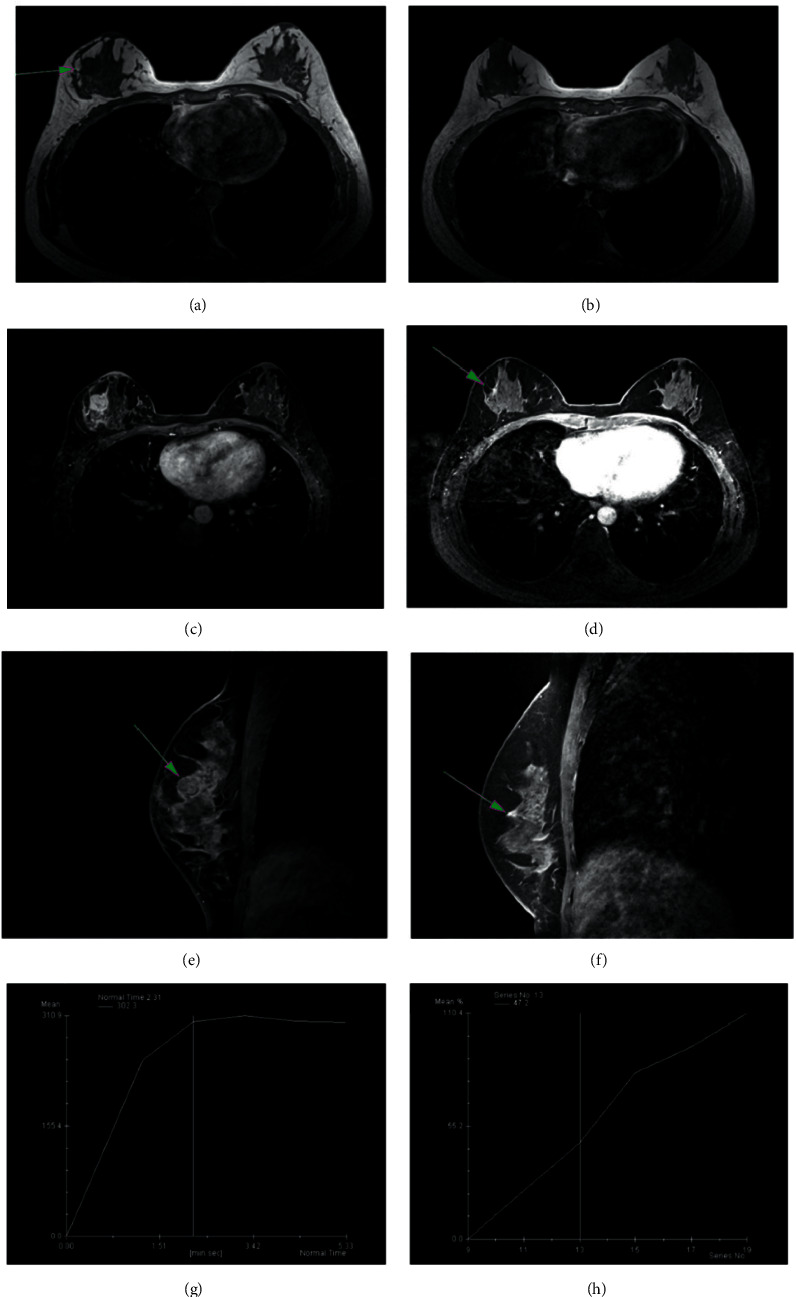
MRI image of triple-negative breast cancer. (a) Before NAC, T2W; (b) after NAC, T2WI; (c) before NAC, T1W1; (d) after NAC, T1W1; (e) before NAC, T1W1; (f) after NAC, T1W1; (g) before NAC, the TIC type; and (h) after NAC, the TIC type is a type I of progressive enhancement.
